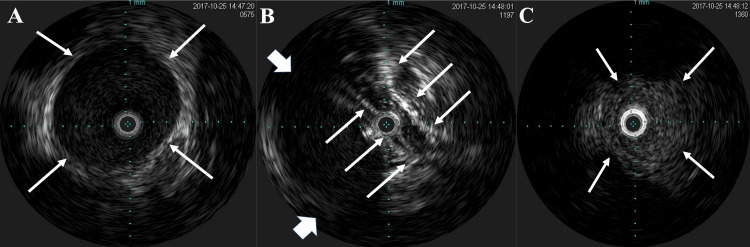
Intravascular ultrasonography. A: Intravascular ultrasonography showing normal structure of the common iliac vein proximal to the obstruction. White arrows delineate the wall of the common iliac vein. B: Intravascular ultrasonography image at the site of obstruction (white arrows) showing that the right external iliac vein was compressed and obstructed by the right external iliac artery (white arrowhead). C: Intravascular ultrasonography showing heavy spontaneous echo contrast distal to the obstruction, suggesting a thrombus. The white arrows indicate the wall of the right external iliac vein.
